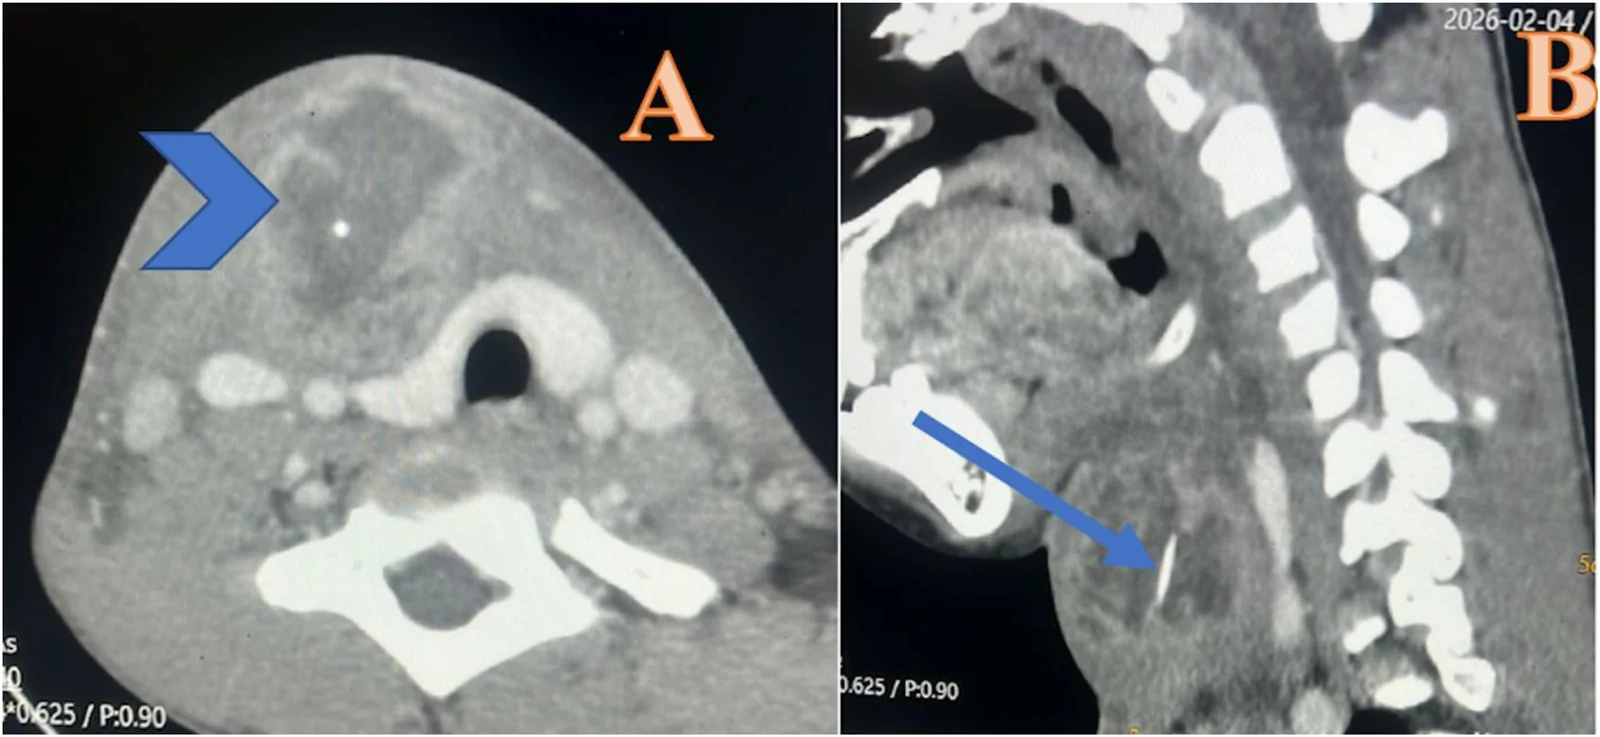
Axial (A) and sagittal reconstructed (B) contrast-enhanced CT images of the cervical region showing a hypodense fluid collection with peripheral rim enhancement (blue arrowhead), consistent with an abscess, and a linear hyperdense structure corresponding to the fish bone (blue arrow).
